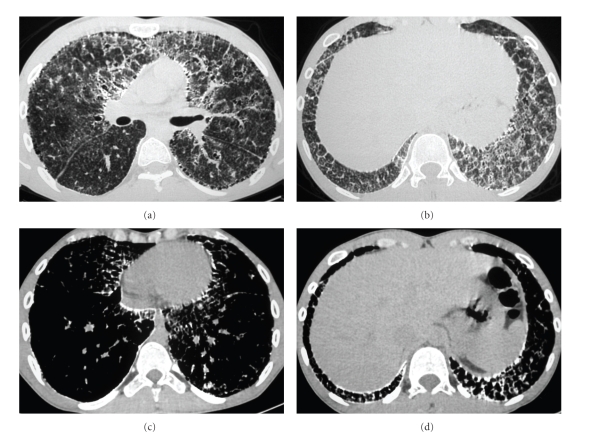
(a-b) High-resolution CT scan reveals diffuse ground glass attenuation and septal thickening, more pronounced in lower pulmonary regions. Note also subpleural cysts. (c-d) Mediastinal window scans shows calcifications along the interlobar septa and subpleural regions.
